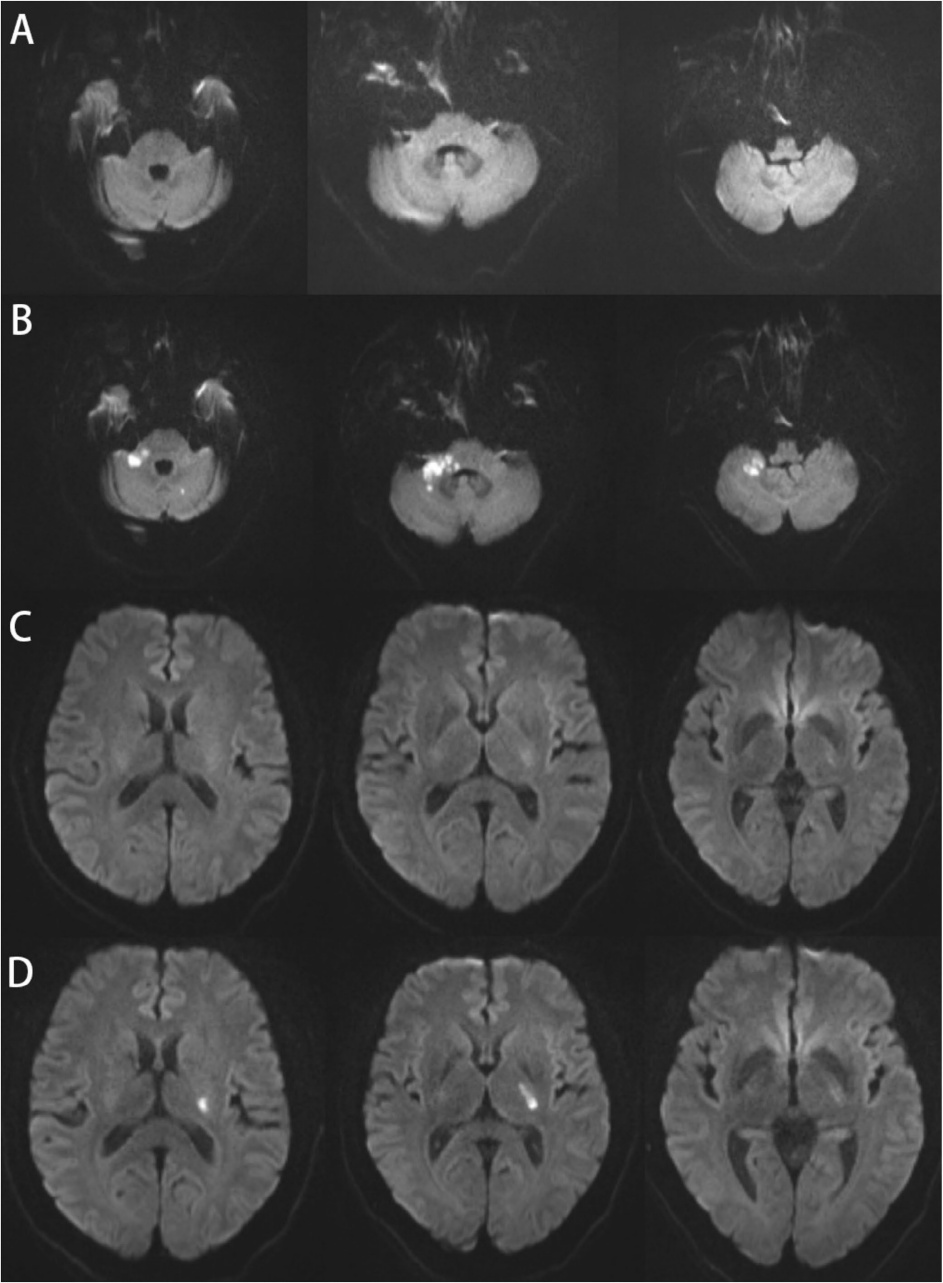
Patients with delayed DWI lesions on follow‐up MRI. (A, B) A patient presented with acute onset vertigo lasting one day. Initial magnetic resonance imaging (MRI) showed no acute ischemic lesions. Follow‐up MRI on day 7 after symptom onset revealed hyperintensity on diffusion‐weighted imaging (DWI) in the bilateral cerebellum and right cerebellar peduncle, confirming an acute ischemic stroke. (C, D) A patient presented with dizziness lasting 22 h. Initial MRI showed no ischemic lesions. Follow‐up MRI one week after admission demonstrated a DWI lesion in the left basal ganglia.
